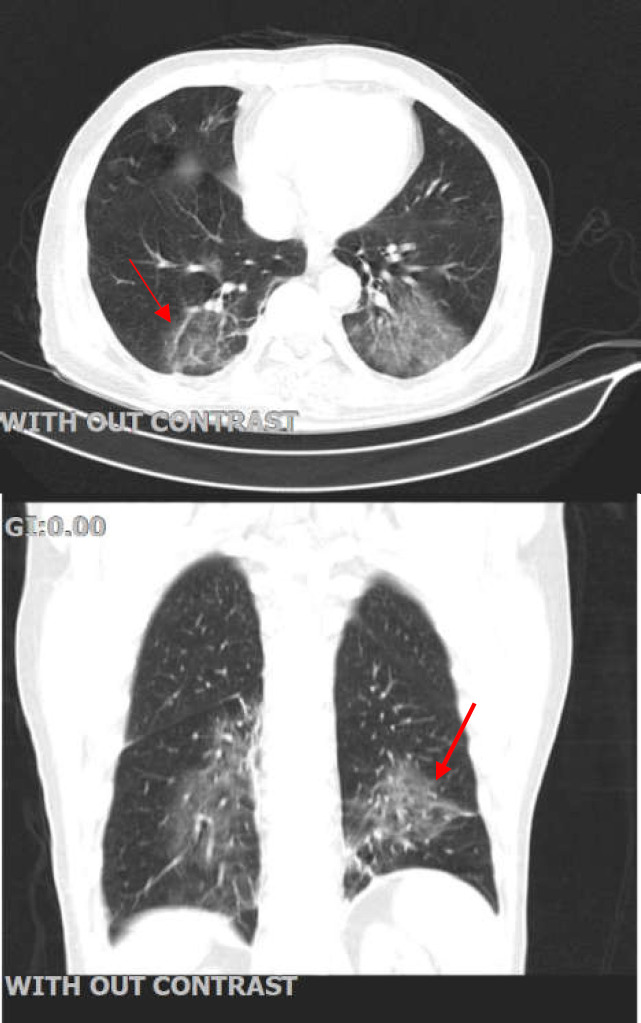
Chest CT scan of the patient. On the chest CT scan, bilateral infiltrations are evident
